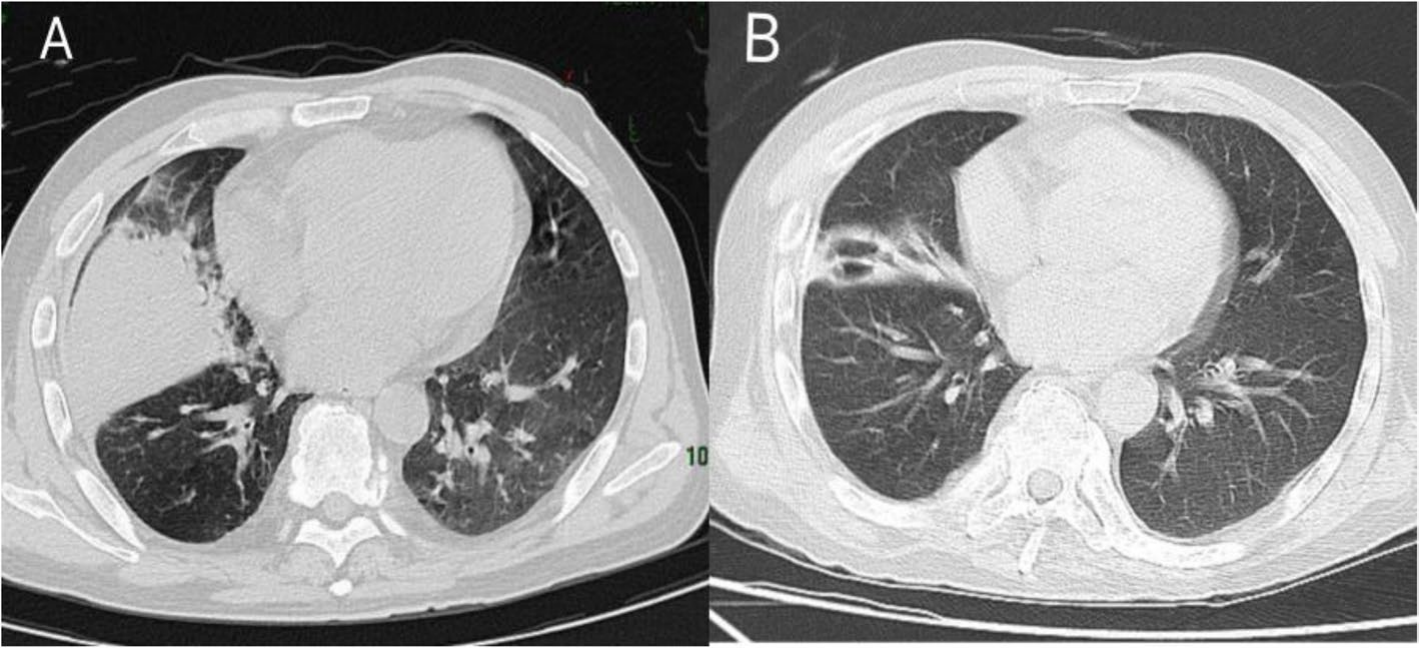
Chest computed tomography (CT) scan in the early stage showed right lower lobe consolidation, multiple calcifications in the bilateral perihilar, and small mediastinal lymph nodes A. Inflammation of the right lung was mostly cleared after antibiotic treatment (B)
